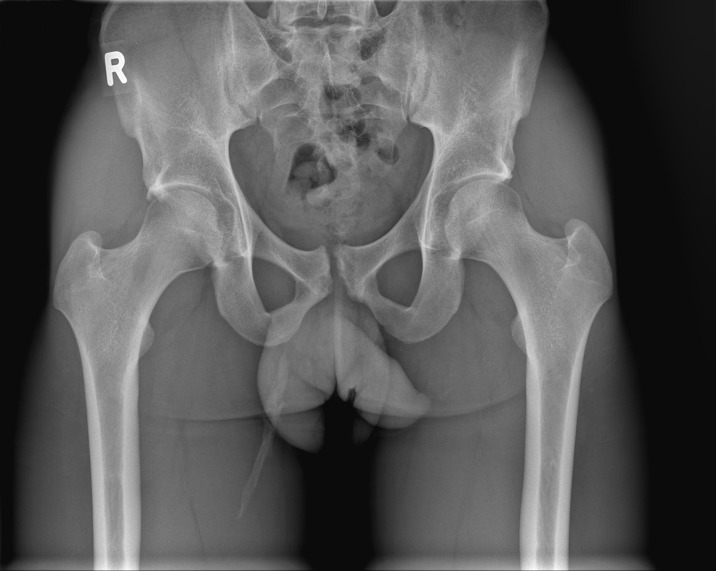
Pelvic X-ray showing calcification in the adductor region.
